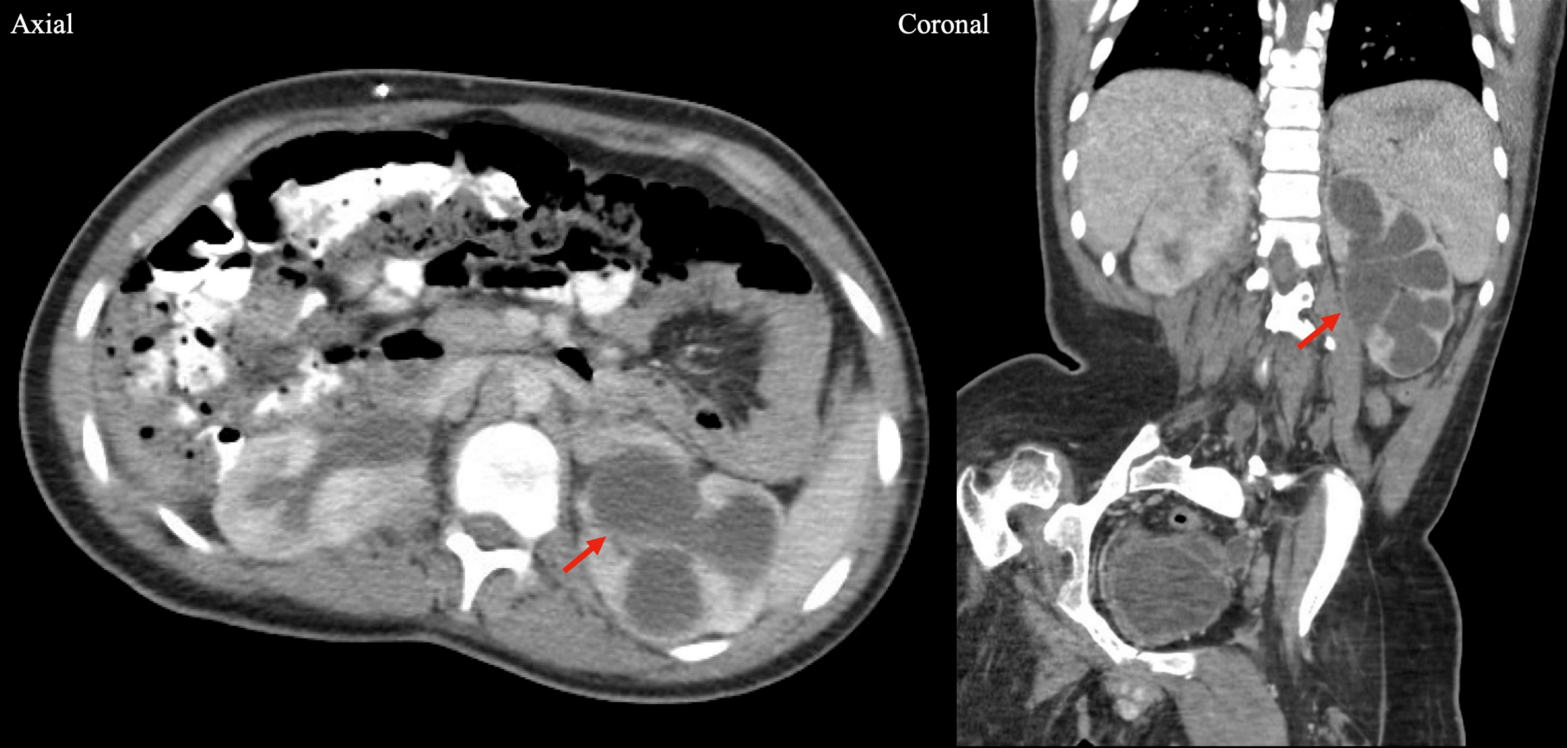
Axial and coronal CT scan of the abdomen and pelvis Axial and coronal CT scan of the abdomen and pelvis, demonstrating left-sided hydronephrosis characterized by dilation of the renal pelvis and calyces (arrows), with associated diminished parenchymal attenuation concerning for early pyelonephritis. CT, computed tomography
